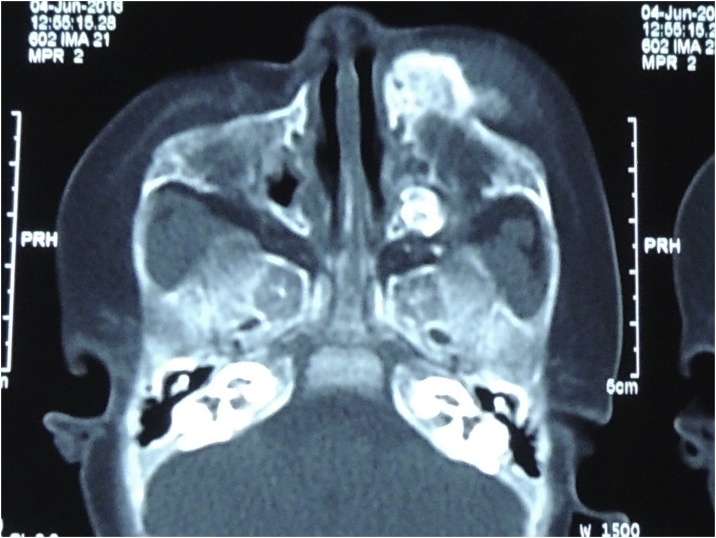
Preoperative Computed Tomography (Axial cut) showing a poorly-defined radiopaque lesion of the left maxilla.
